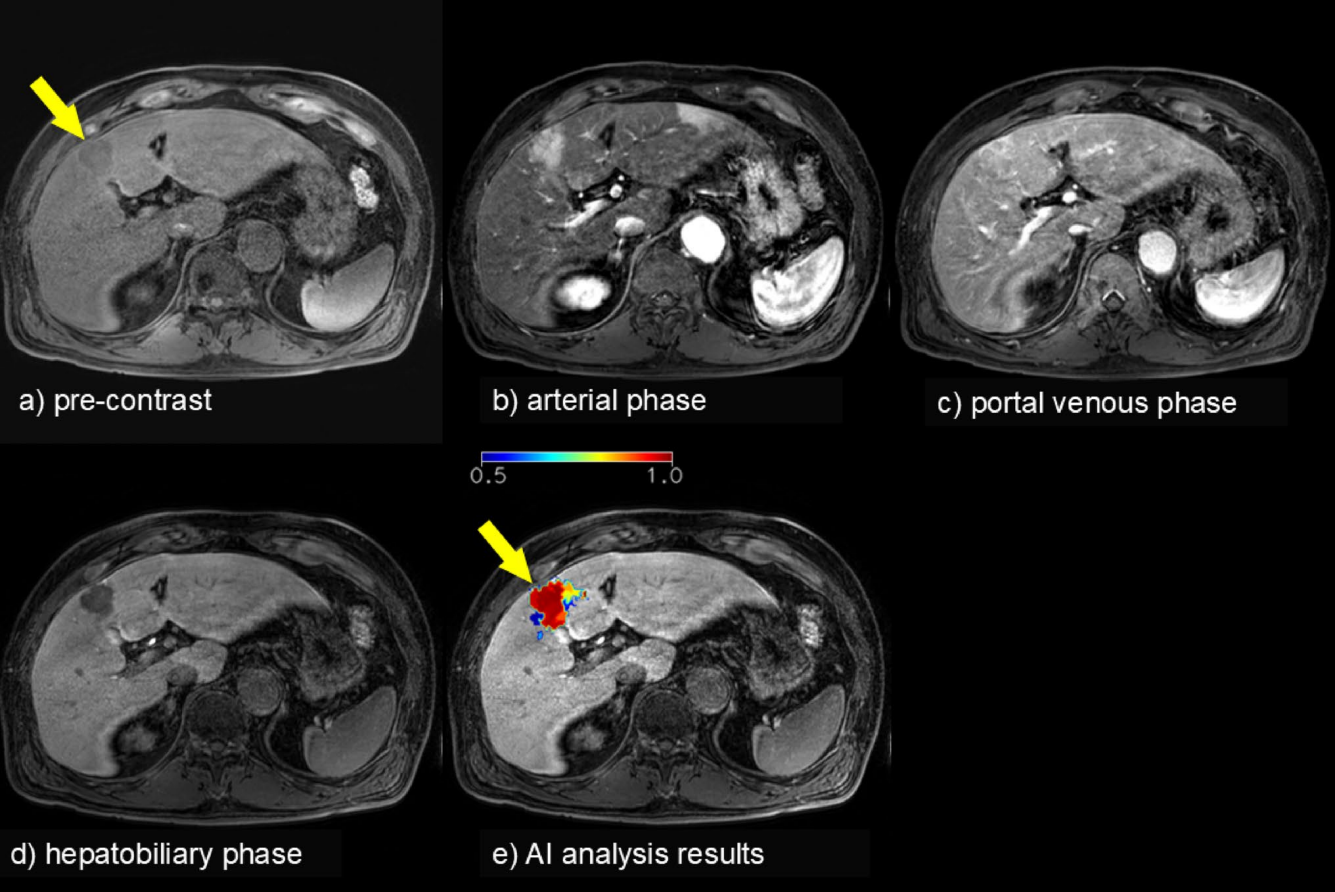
Example of a process using Gd-EOB-DTPA-enhanced MRI reading support software with AI. A 35-mm tumor is identified in the medial segment (a: arrow). This hypervascular tumor was diagnosed as HCC. The MRI reading support software (Cal.Liver.Lesion; Bayer Yakuhin Ltd., Osaka, Japan) quantitatively scores the degree of signal variation compared to surrounding tissue. The score, displayed in color, is overlaid on the hepatobiliary phase image, where the HCC region exhibits high values (red) (e: arrow). Although not FDA-approved, the software received regulatory approval for clinical use in Japan; however, it has been discontinued as of 2025. Assistance from this AI software could enhance the accuracy of MRI-based liver tumor diagnosis. *AI* Artificial intelligence, *Gd-EOB-DTPA* Gadoxetic acid, *HCC* Hepatocellular carcinoma, *MRI* Magnetic resonance imaging
